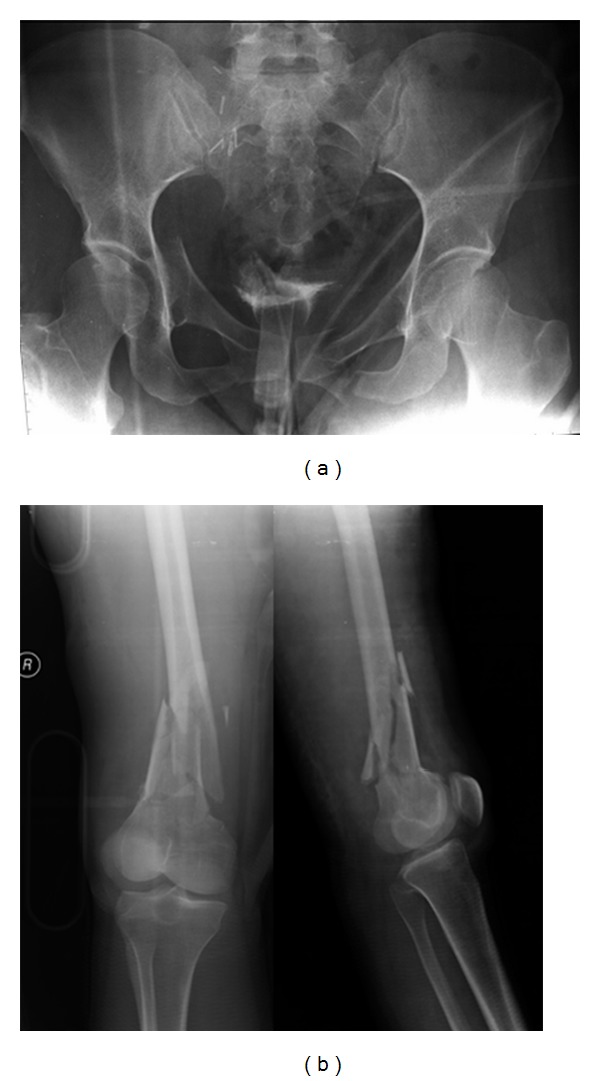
X-ray in the emergency room showing (a) the right-sided anterior column and the left-sided pubic rami fractures of acetabulum. (b) The right-sided distal femoral metaphyseal complex (OTA 33-D3) fracture.
